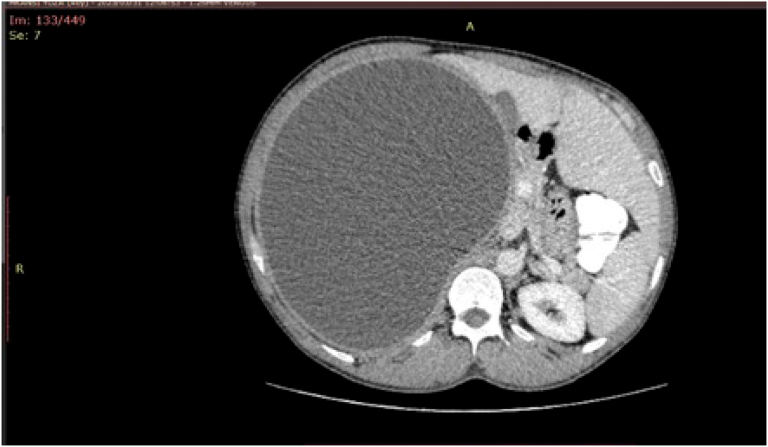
Initial CT scan of the abdomen.
